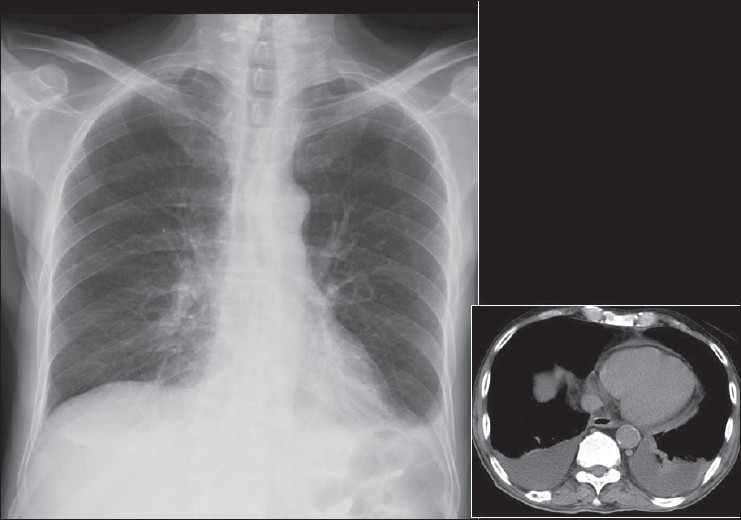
The chest radiograph and CT scan were taken on the same day, 7 hours apart. There is nothing suspicious on the chest radiograph to suggest a pericardial effusion. There is blunting of the left costophrenic angle, suggestive of a small pleural effusion. However, the axial CT scan shows a small pericardial effusion and moderate bilateral pleural effusions
